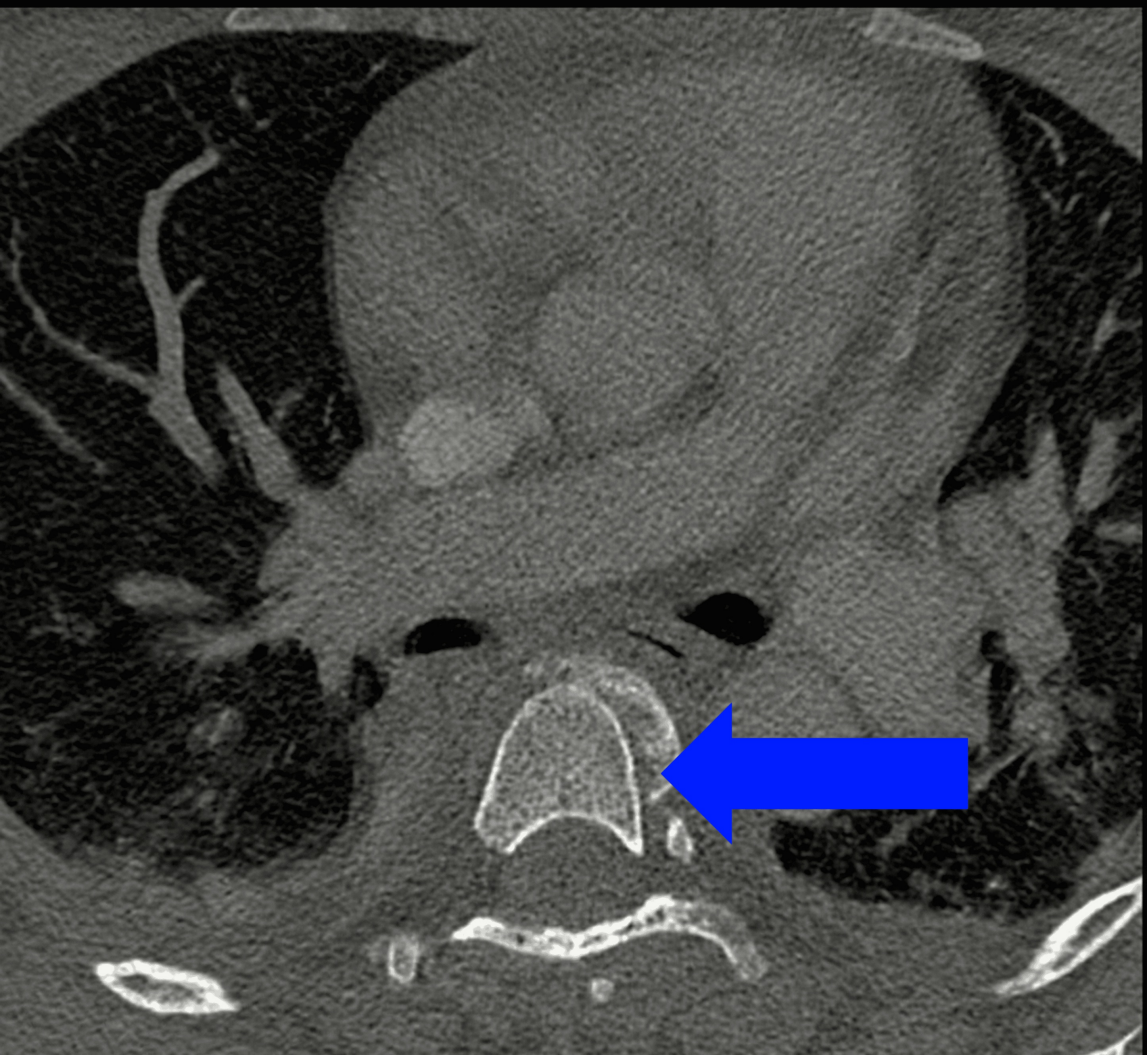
Axial CT scan of the thoracic spine Axial CT scan showing a fracture-dislocation at the level of T4-T5.
